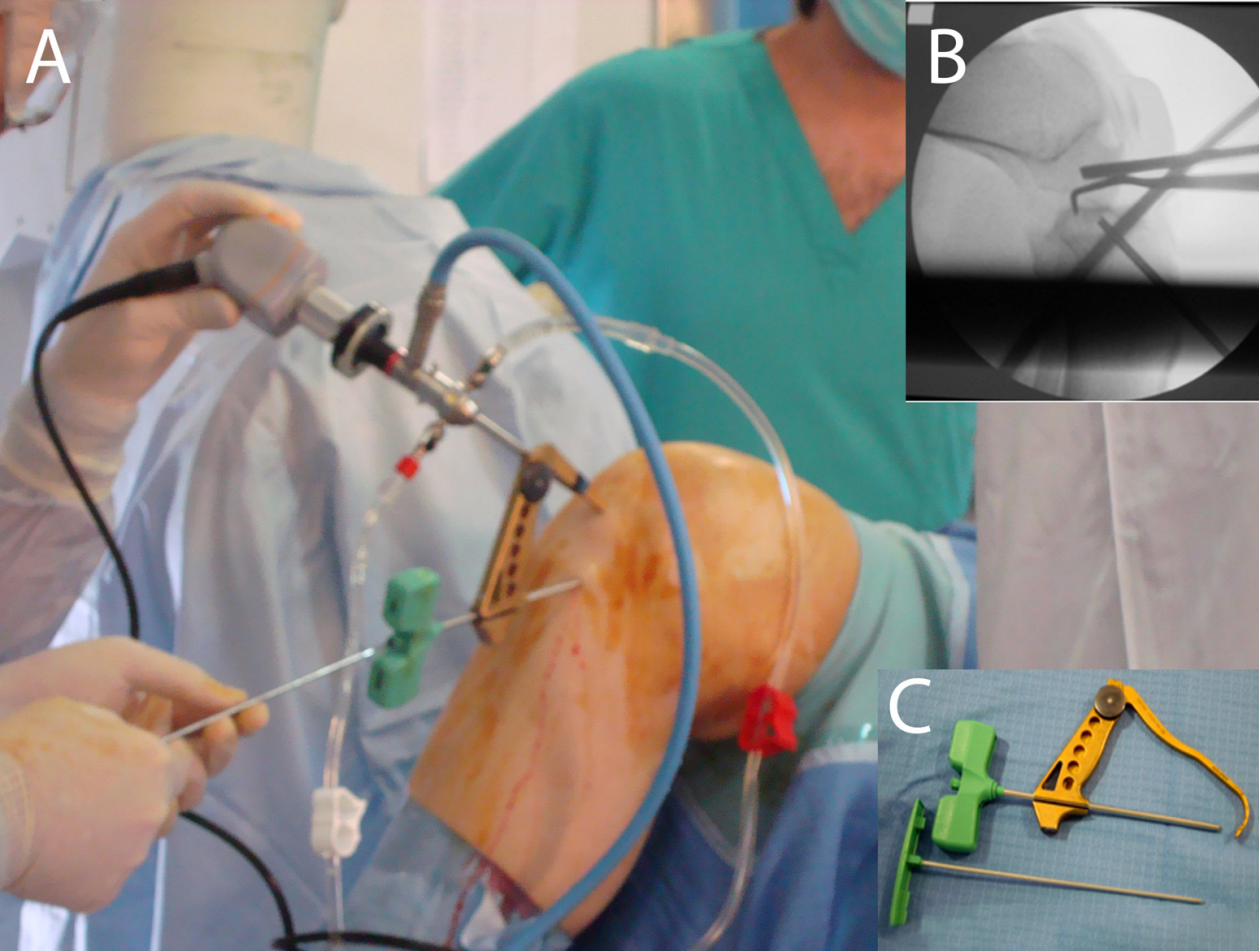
A: The biopsy is performed through the access usually used to reconstruct the anterior cruciate ligament. B: X-rays intensifier Check showing the correct placement of the biopsy trocar. C: the biopsy trocar and the guide used.
